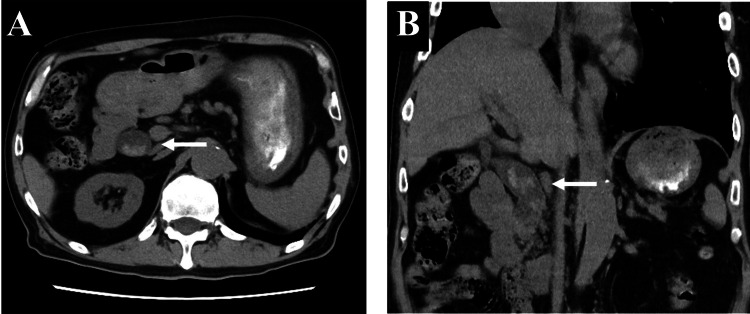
Abdominal computed tomography after the recurrence of epigastric pain. A high-density lesion was detected in the extended extrahepatic bile duct (arrow) in the (A) axial and (B) coronal sections.
